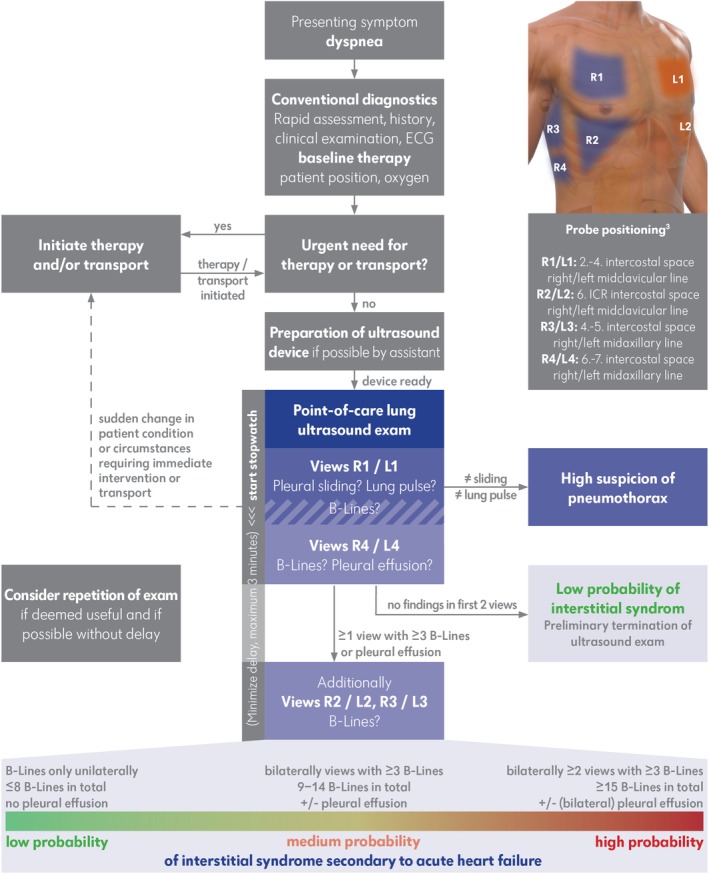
Algorithm for pre‐hospital point of care ultrasound for patients with dyspnea.
